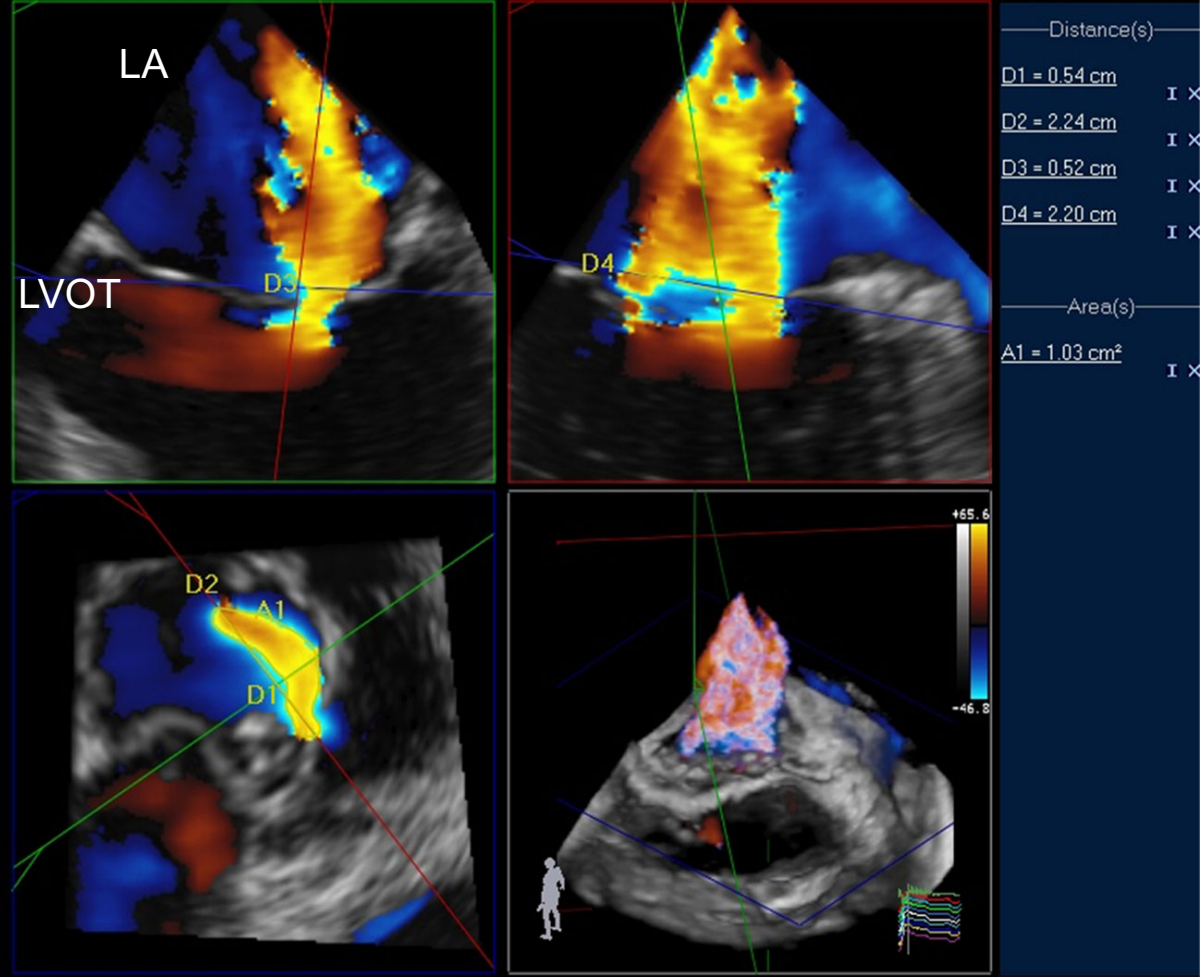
RT3D color Doppler TEE dataset in a patient with severe functional MR. Display of 3D analysis software (Qlab 9.0, Philips Medical Systems) showing a 3D view to the mitral valve and the MR jet from an LA perspective (*bottom right*) and three reconstructed image planes in orthogonal orientation to the MR jet: long-axis LVOT view (*top left*), 2-chamber view (*top right*), short-axis view showing the asymmetric VCA (1.03 cm^2^ by direct planimetry, short-axis diameter (*D1*) = 0.54 cm, long-axis diameter (*D2*) = 2.24 cm) along the commissure line (*bottom left*). *LA* left atrium, *LVOT* left ventricular outflow tract
